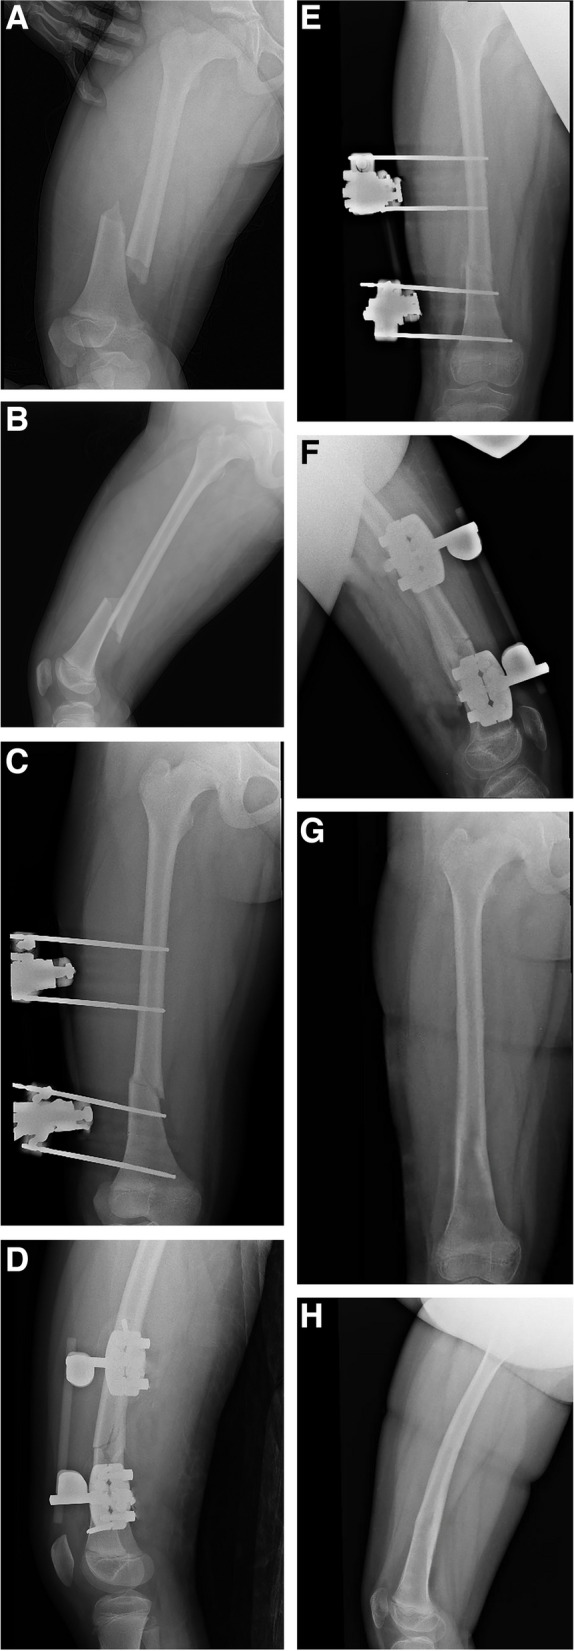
A 9-year-old girl with distal MDJ fracture caused by motor vehicle accident. Preoperative radiography showed complete displacement of the fracture with significant shortening (A, B); the fracture was treated with EF, and postoperative X-rays showed satisfactory reduction (C, D); with the fracture union after 3 months (E, F), the implant was removed under sedation, and the range of motion of the knee joint and lower limb function were normal at the final follow-up (G, H)
